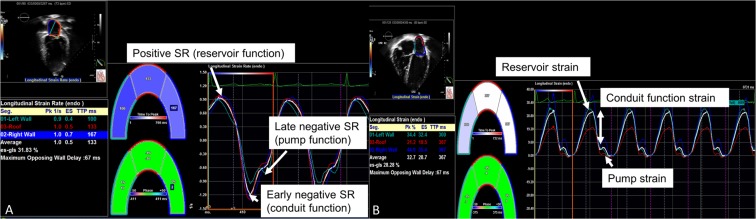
Atrial strain and strain rate by echocardiogram. (A) represent atrial strain curves measured over the cardiac cycle. The white curve represents average atrial strain curve. (B) represents atrial strain rate curve.
